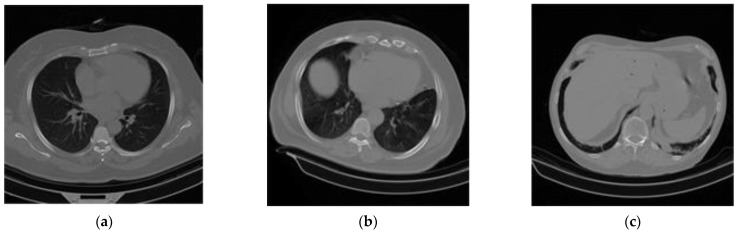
A sample of the experimental CT scan dataset: (a) normal or non-COVID-19, (b) COVID-19, and (c) community-acquired pneumonia (CAP).
